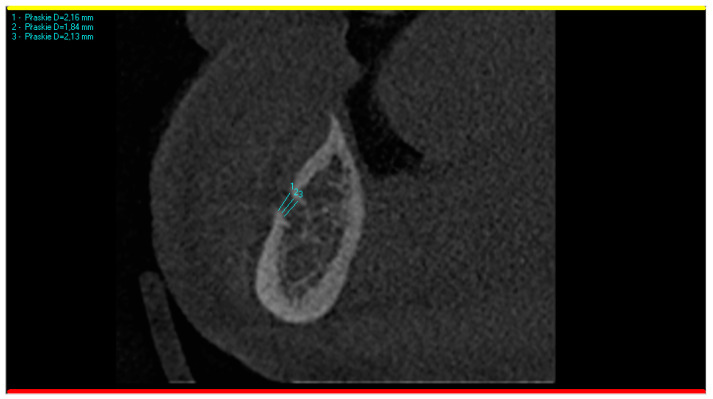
CBCT scan showing the methodology of measuring the mental foramen. Three measurements were taken (results 2.16, 1.84, and 2.13 mm are visible in the upper left corner) and then the mean diameter was calculated (2.04 mm) to obtain the most reliable result.
